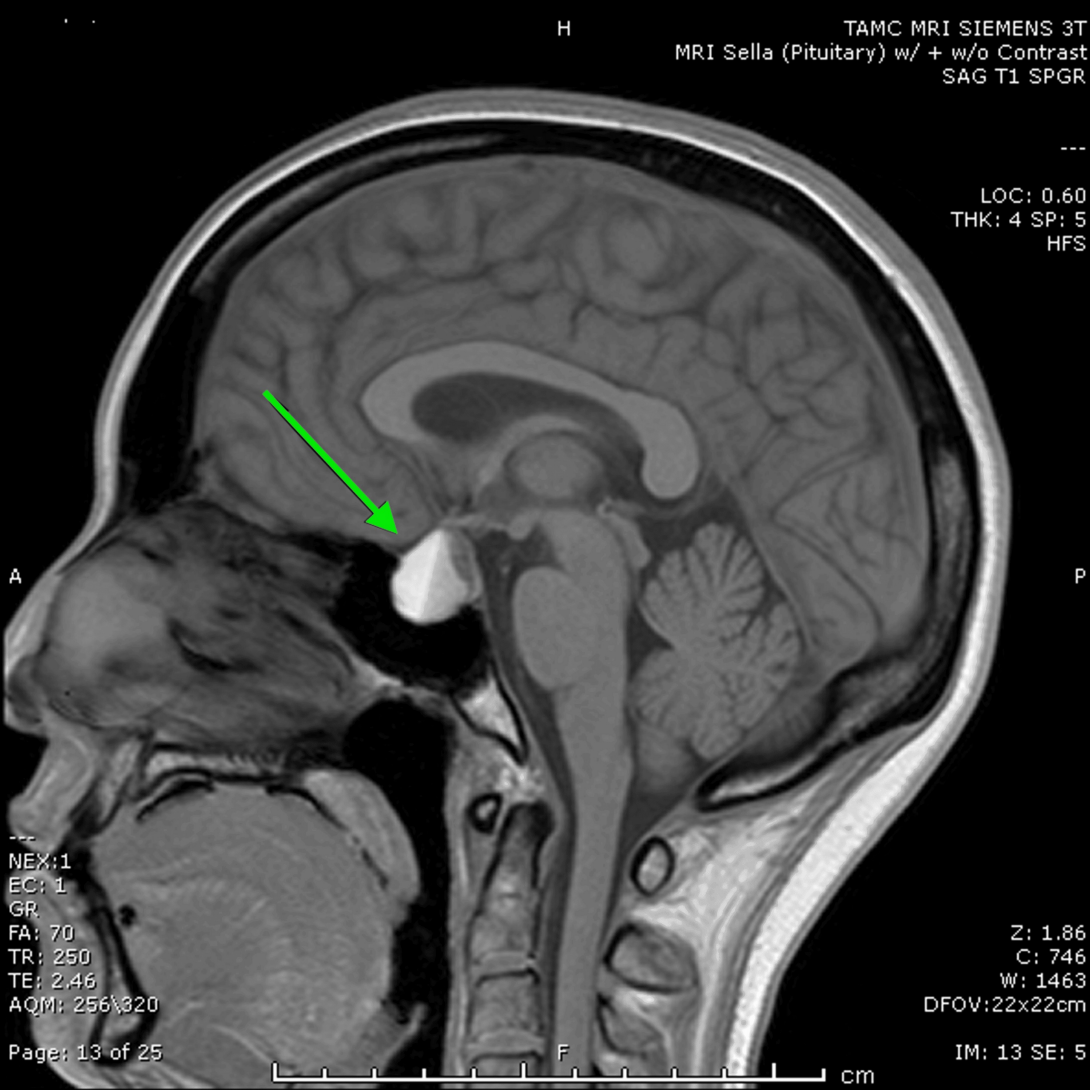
Sagittal T1-weighted MRI image demonstrating a large pituitary mass with internal hemorrhage, exerting mass effect on the optic chiasm. Note the demarcation indicating the fluid-fluid level, with the hyperintense region representing acute to subacute bleed and the hypointense region likely representing serous or proteinaceous fluid.
